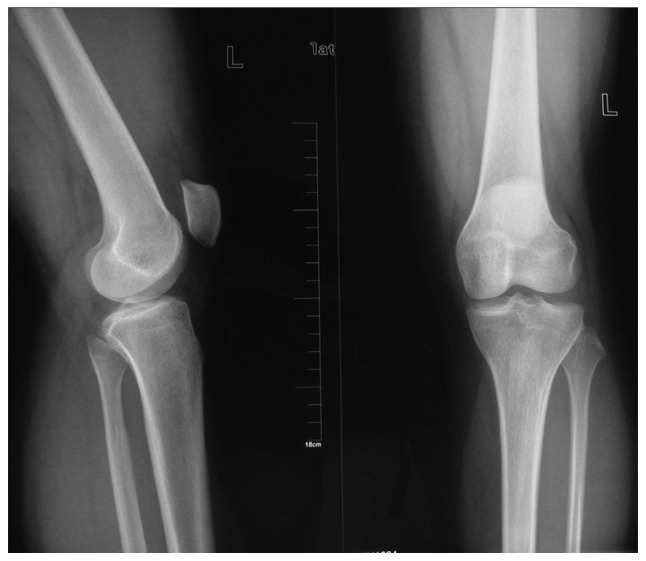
X-ray imaging prior to surgery shows a widened lateral joint space.
